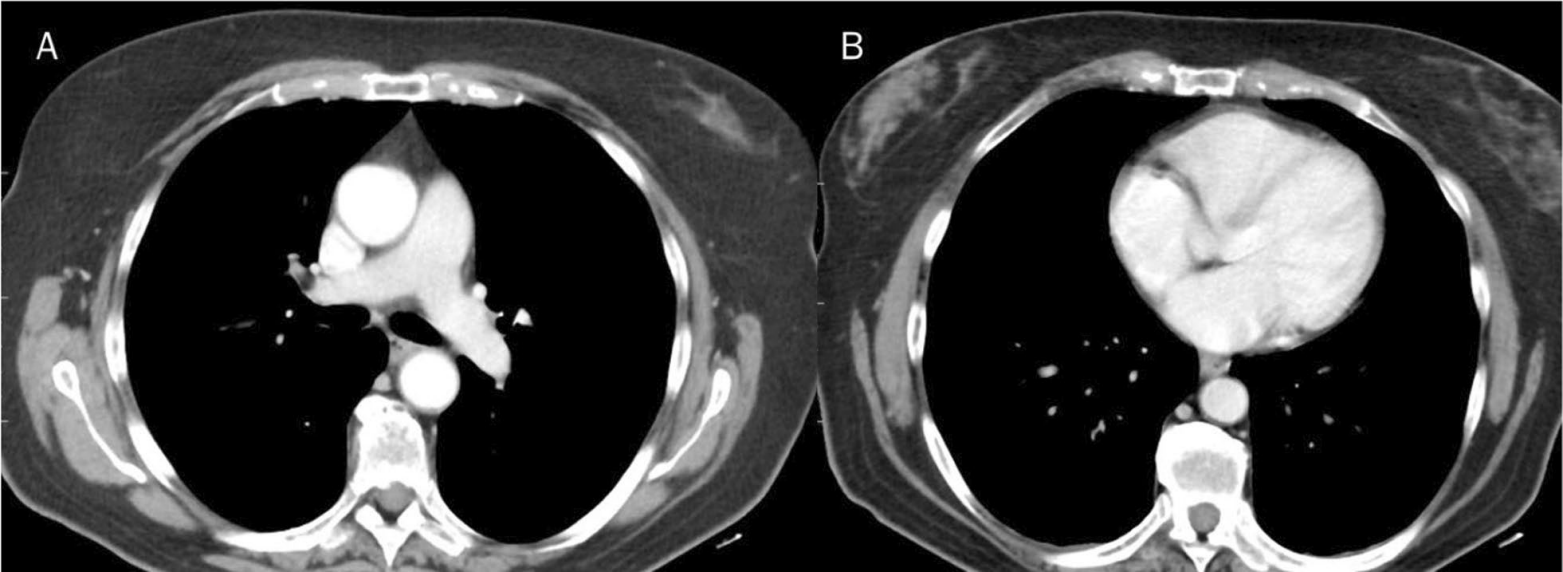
Computed tomography study do not identify the presence of lymphadenopathy in the axillary area and lymphangitis (A, B)
